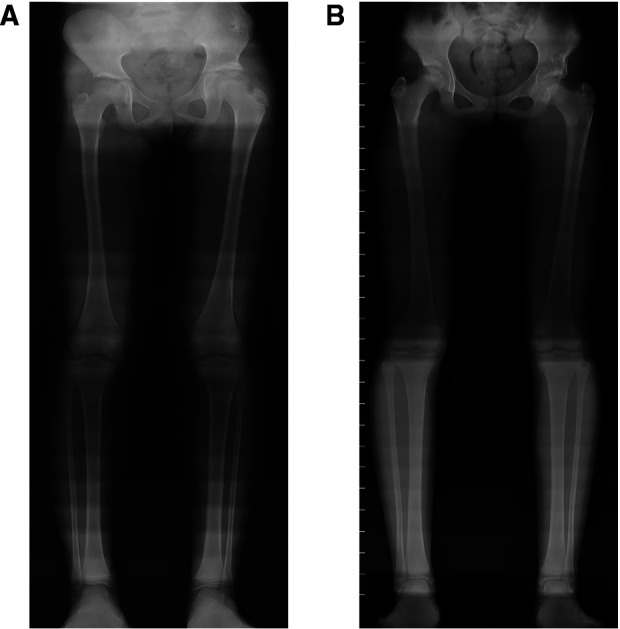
Two cases of LLD after pelvic osteotomy combined with femoral shortening osteotomy (A) full-length lower extremity weight-bearing radiographs obtained 50 months after the Salter pelvic osteotomy combined with femoral shortening osteotomy. The length of left and right femur was 350 mm and 343 mm respectively. The whole length of the left and right lower limb was 647 mm and 638 mm respectively. (B) Full-length lower extremity weight-bearing radiographs obtained 47 months after the Pemberton osteotomy combined with femoral shortening osteotomy. The length of left and right femur was 378 mm and 391 mm respectively. The whole length of the left and right lower limb was 706 mm and 721 mm respectively.
